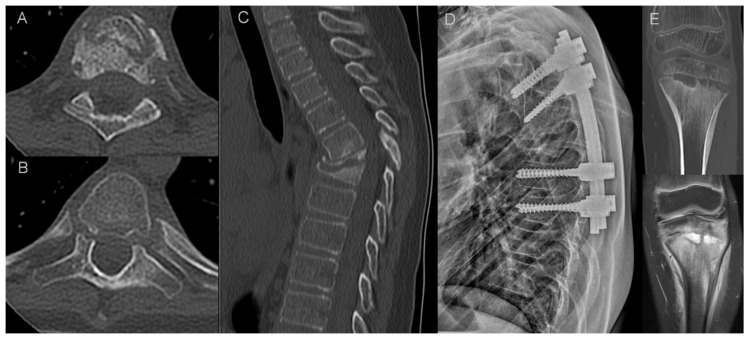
Imaging findings in a 10-year-old patient with CRMO (patient 16.). Axial CT scans show erosions in the facet (A) and costovertebral (B) joints. Sagittal CT reformat shows osteodestructive lesions with vertebra plana deformity (C) that led to progressive kyphosis with spinal stenosis requiring transpedicular stabilization (D). Another site of the disease was found in the tibia (E) with CT and MRI showing metaphyseal osteolysis with adjacent osteosclerosis, extensive bone marrow edema and solid periosteal reaction.
